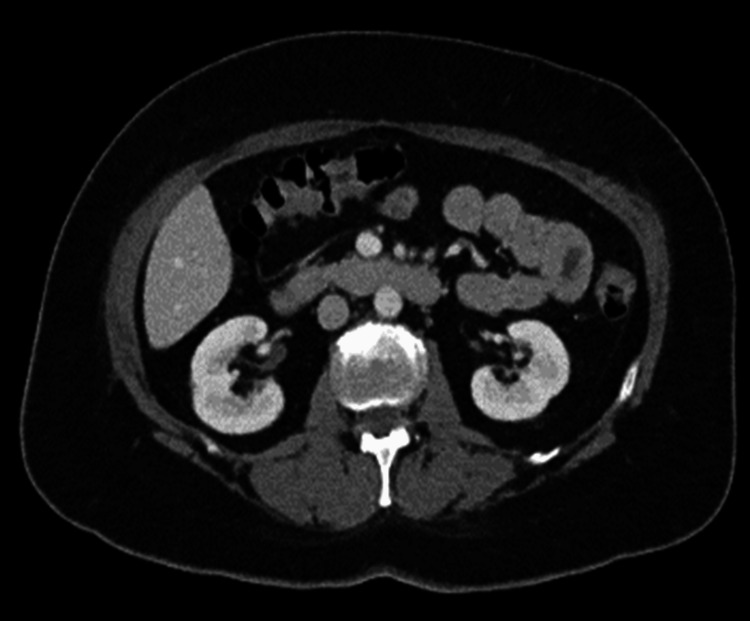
Computed tomography scan on recent admission showing no acute intraabdominal process with no inflammatory changes of the colon. General evidence of cholelithiasis, hepatic steatosis, and colonic diverticulosis were noted.
